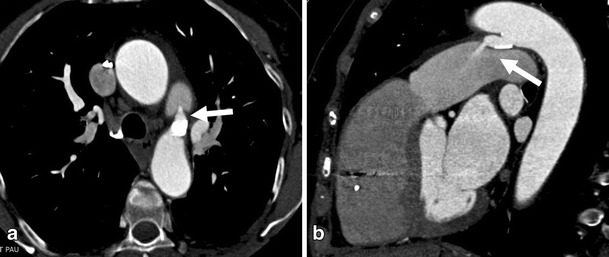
A 61-year-old female patient visited the ED with chest pain and mild dyspnoea. The patient was diagnosed with atrial fibrillation and had carried a pacemaker since 2000. Echocardiography showed a questionable patent ductus arteriosus (PDA). A thoracic CTA was ordered. Patent ductus arteriosus. (a) CT axial image shows a tubular structure (arrow) abutting aorta with adjacent coarse calcification. (b) Sagital multiplanar reconstruction shows enhanced blood flowing from aorta to pulmonary artery via patent ductus arteriosus
